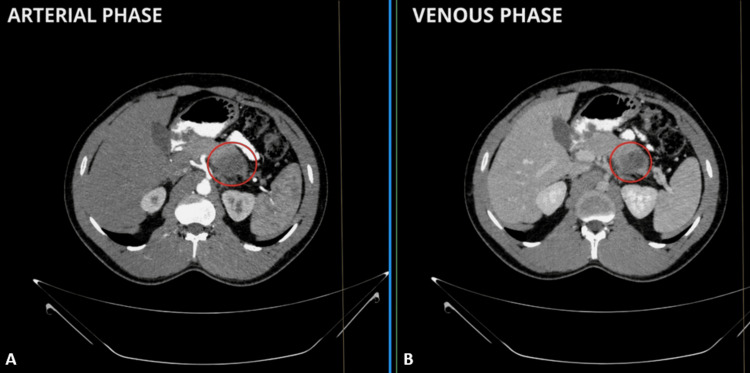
Arterial (A) and venous (B) phase contrast-enhanced CT images demonstrating a hypoattenuating lesion in the tail of the pancreas (circled in red) The lesion is better characterized on venous phase imaging.
